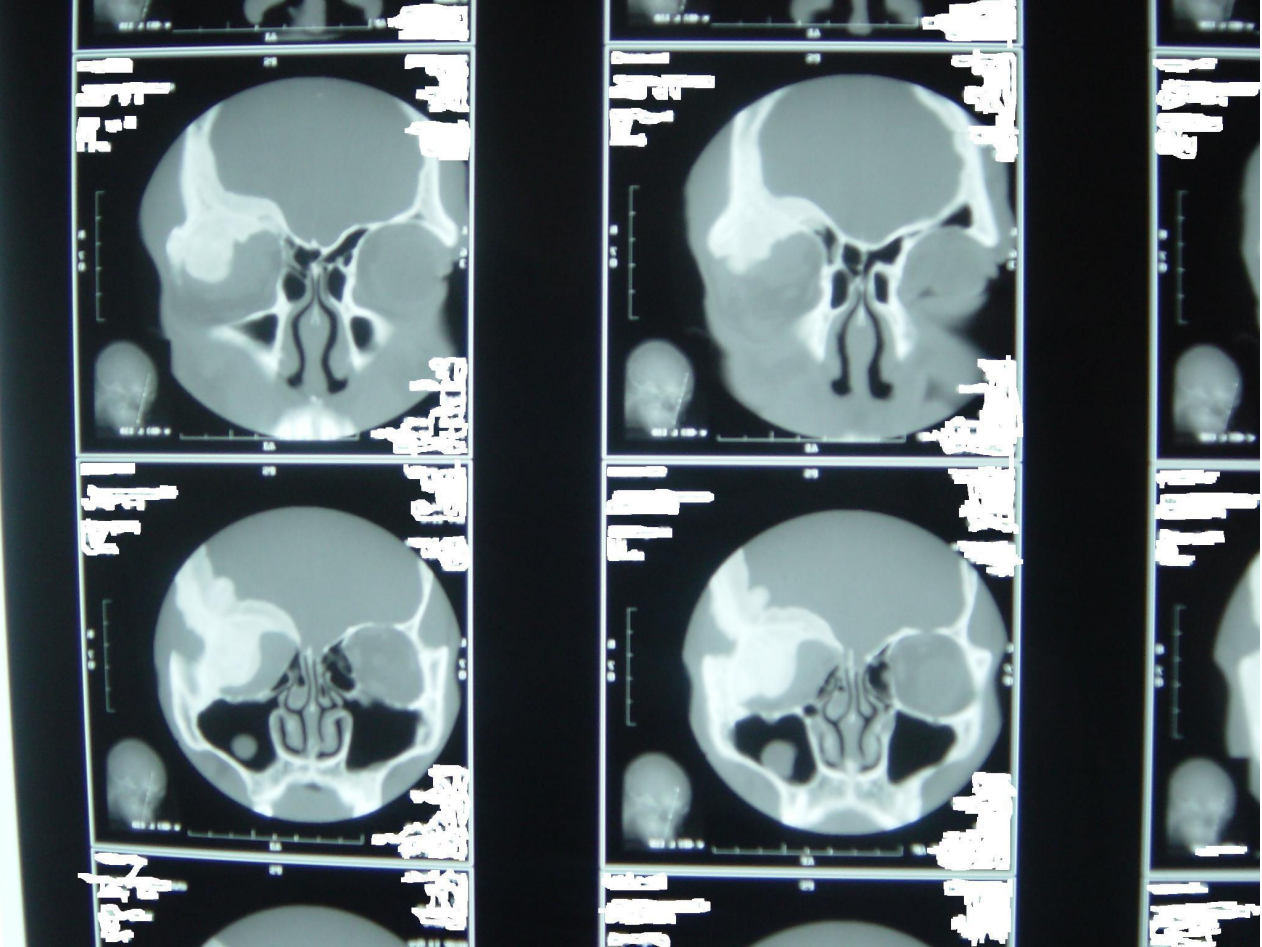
Preoperative cranial computed tomography scan showing expansile bony mass of the right orbit involving the greater wing of the sphenoid, the medial wall of the orbit and the greater wing of the sphenoid (frontal view). There is encroachment into the retro-orbital space with obvious proptosis of the right globe. There is no evidence of intracranial extension.
